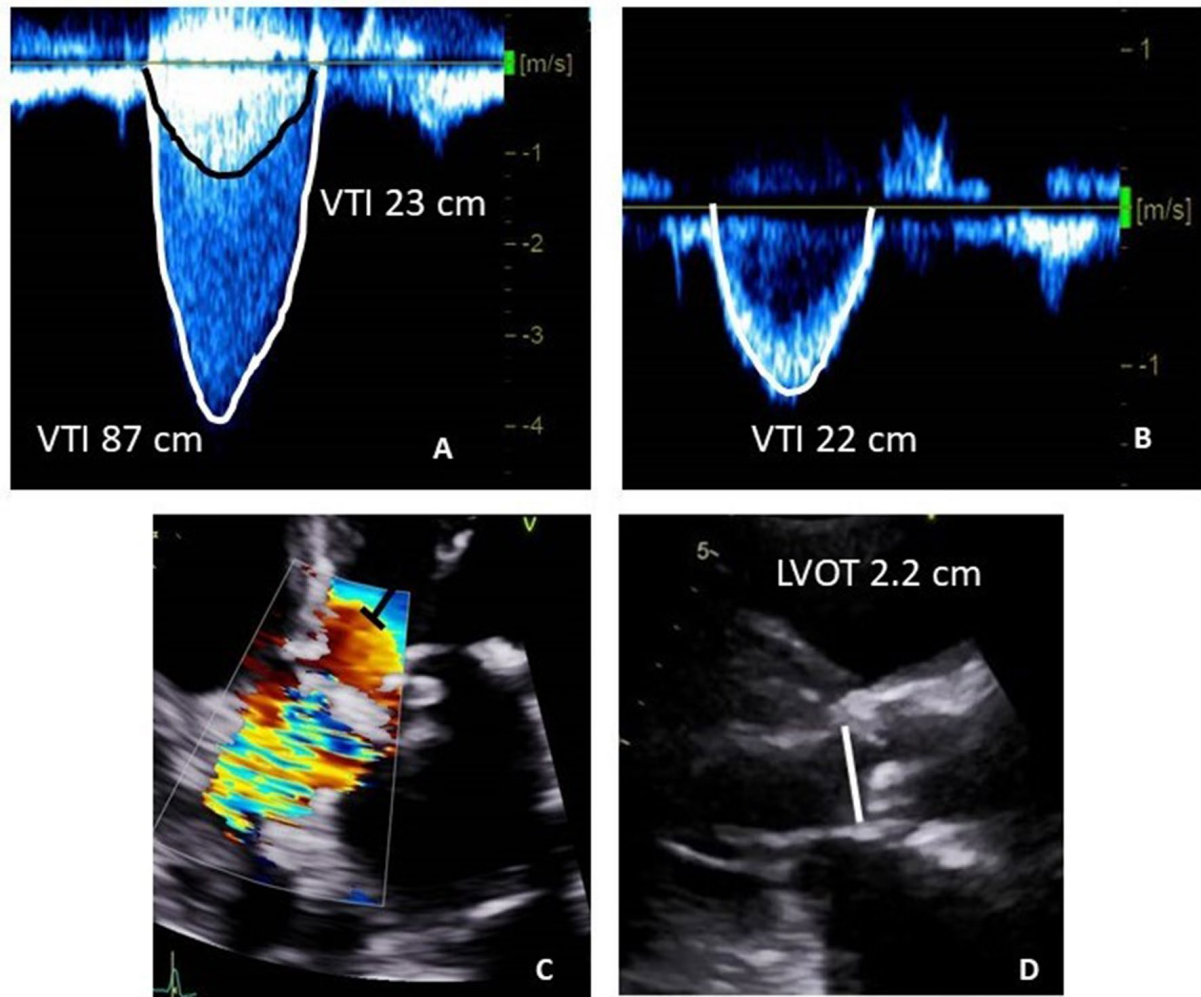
Examples of the using pre-attenuation velocity envelope for aortic valve area calculation. An example of pre-attenuation velocity envelope on CW Doppler recording (panel A) obtained from 5-chamber view, with clearly defined and traced envelopes. PW Doppler derived LVOT velocity from 5-chamber view is shown (panel B). The edge of the flow acceleration zone and recommended PW Doppler sampling area as suggested by guidelines in the 5 -chamber view is shown (panel C). LVOT diameter measurement in the parasternal long axis view is shown (panel D). Assuming the shown CW velocity is the highest obtained transaortic velocity, the aortic valve area using pre-attenuation velocity calculates at 1.00 cm2 and the aortic valve area using the standard PW Doppler calculates at 0.96 cm2
